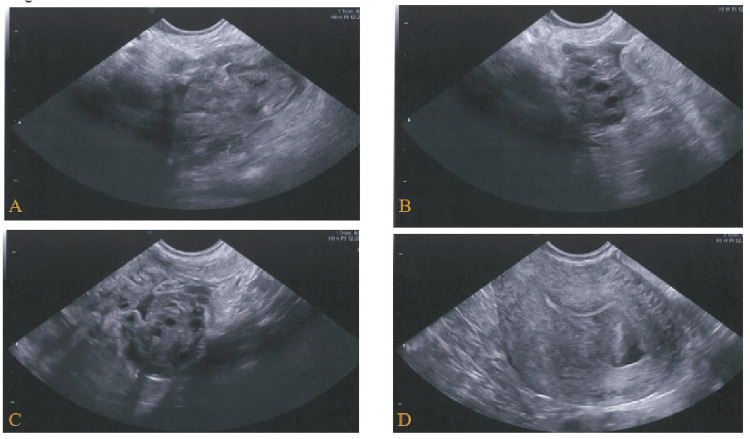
TVUS: transvaginal ultrasonography (A) a heterogeneous left adnexal mass with associated pelvic free fluid; (B,C) preserved bilateral ovarian morphology; and (D) an empty uterine cavity without evidence of intrauterine gestation.
